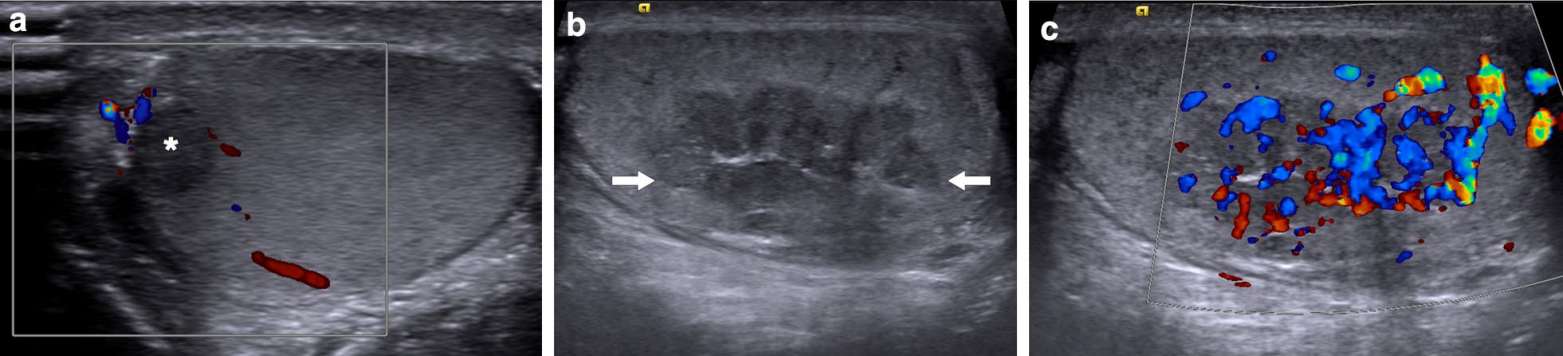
Two different patients with known history of congenital adrenal hyperplasia. a Gray scale and color Doppler US images show round lesions in both testes in 13-year old male. The most prominent lesion appears as a focal hypoechoic lesion within the mediastinum of the right testis (asterisk). There was no significant internal vascularity on color Doppler US. Based on the patient’s history and imaging findings, testicular adrenal rests was considered. Serial US exams over 2-years confirmed stability of testicular lesions. b-c Gray scale US b shows bilateral lobulated, large-sized hypoechoic lesions within the mediastinum testis in 15 years-old male. The largest lesion was detected within the right testis as a predominantly hypoechoic, heterogenous lesion (arrows). c On color Doppler US, there was intense internal vascularity within the lesion. These lesions were also considered to be consistent with testicular adrenal rests
